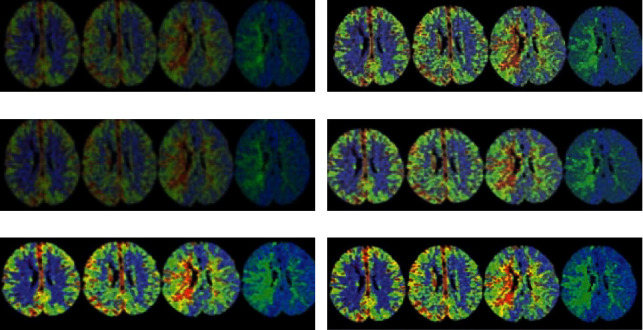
The processing results of CT image under different reconstruction methods. (a) A-FBP. (b) B-FBP. (c) B-iDose4. (d) B-IMR1. (e) B-IMR2. (f) B-IMR3.
